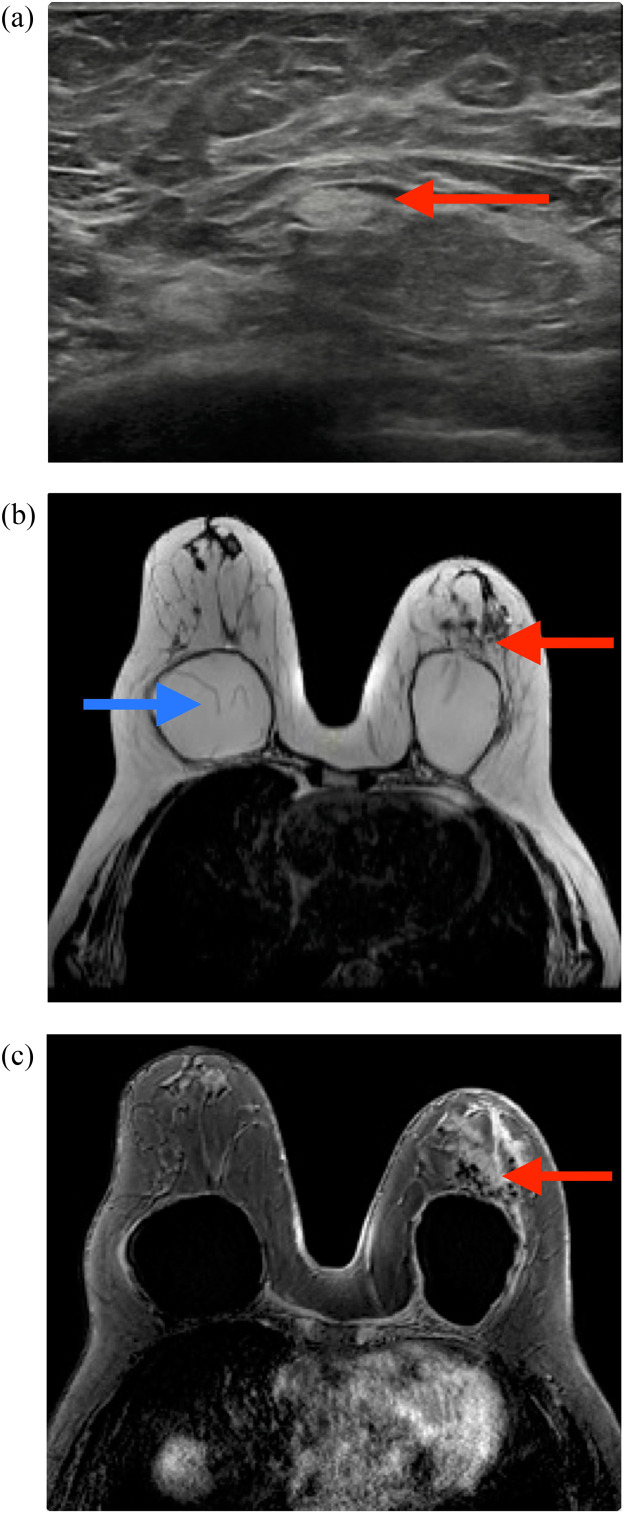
Imaging of breast US and MRI exam of a patient with SBI rupture
